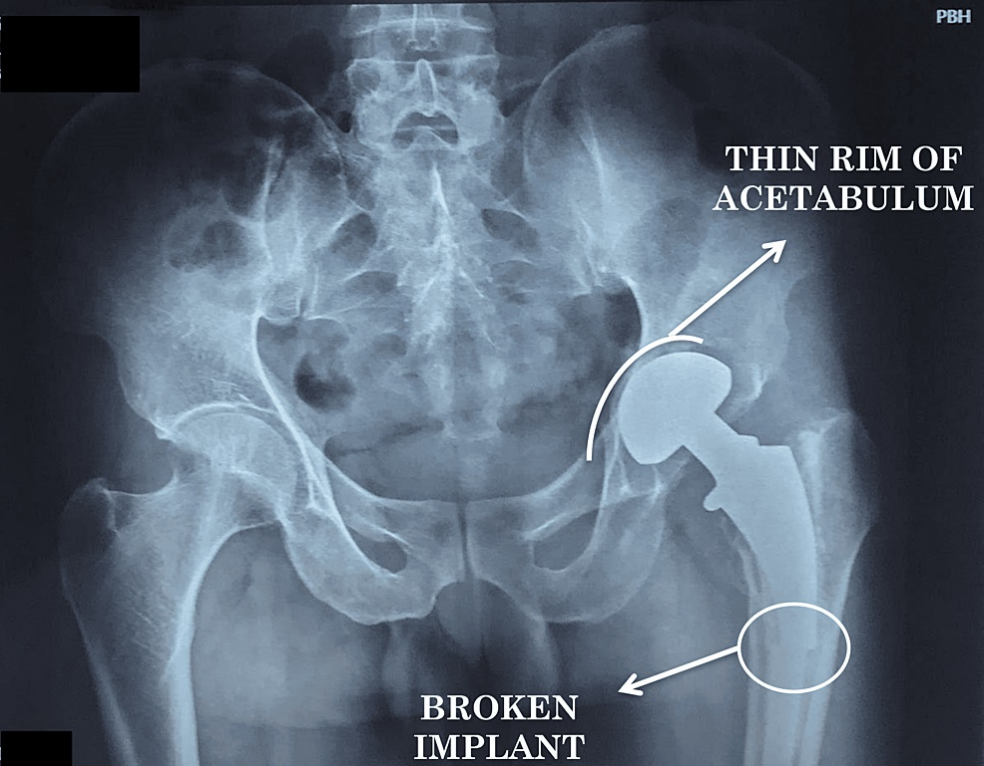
Plan radiograph of the pelvis with both hips showing the thin rim of the acetabulum and the broken implant
